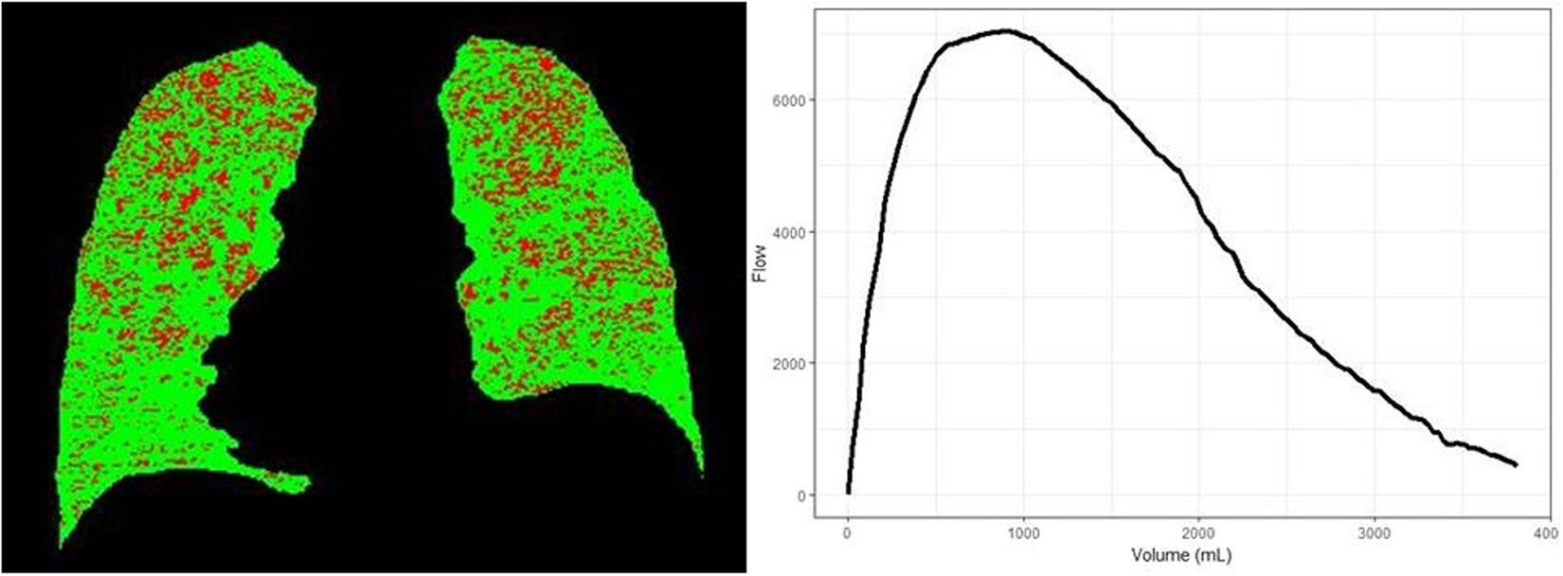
Representative images for a 54 year old African American male with a 34 pack-year smoking history who had significant symptom burden, with mMRC score of 3, and SGRQ score of 48. His lung function by traditional criteria was normal with FEV_1_/FVC of 0.72, and FEV_1%_predicted of 100.1%. Flow volume curve appears normal, however Parameter D was −0.08, which is abnormal. Computed tomography revealed 0.5% emphysema and 25% fSAD (highlighted in red). mMRC = Modified Medical Research Council Dyspnea Scale. SQRQ = St. George’s Respiratory Questionnaire. FEV_1_ = Forced Expiratory Volume in the first second. FVC = Forced Vital Capacity. fSAD = Functional small airway disease.
